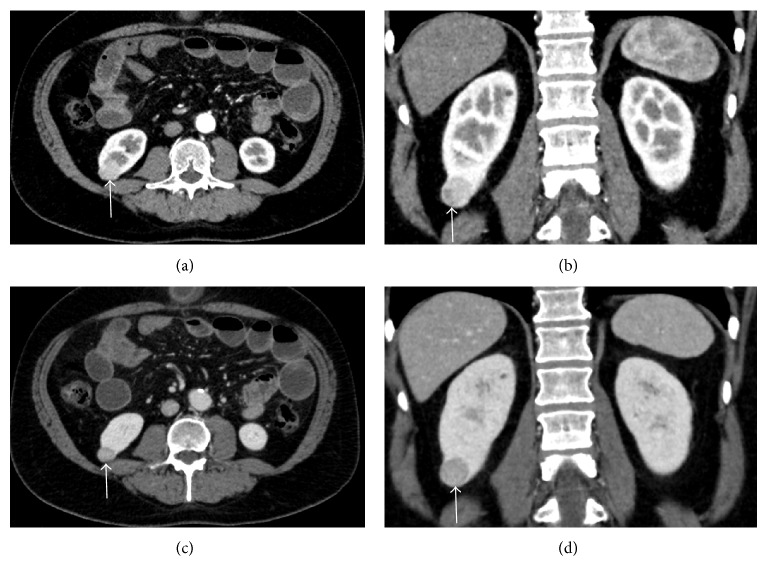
Chromophobe carcinoma. ((a)-(b)) Contrast-enhanced CT scans in arterial phase. ((c)-(d)) Portal phase CT scans. A small tumor with nonspecific morphology is recognizable at the lower pole of the right kidney (arrows).
